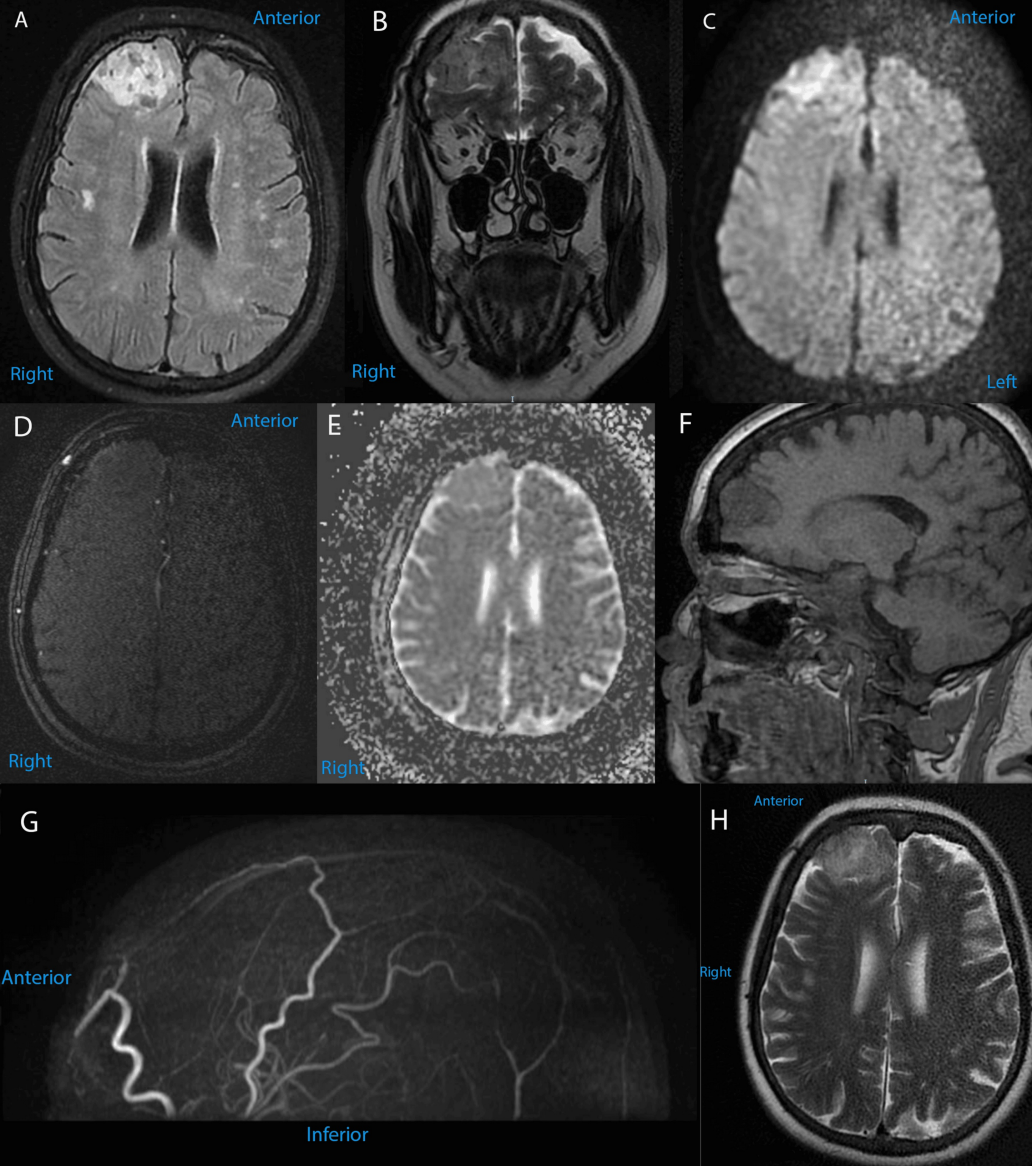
Preoperative MRI brain images of our patient, with different sequences. (A) Axial FLAIR sequence; (B) coronal T2 FSE; (C) axial diffusion-weighted scan (b1000); (D) axial 3D TOF; (E) axial ADC; (F) sagittal 3D T1; (G) sagittal (cross-section) 3D TOF MR angiogram; (H) axial T2 propeller. FLAIR, fluid-attenuated inversion recovery; FSE, fast spin echo; TOF, time-of-flight
